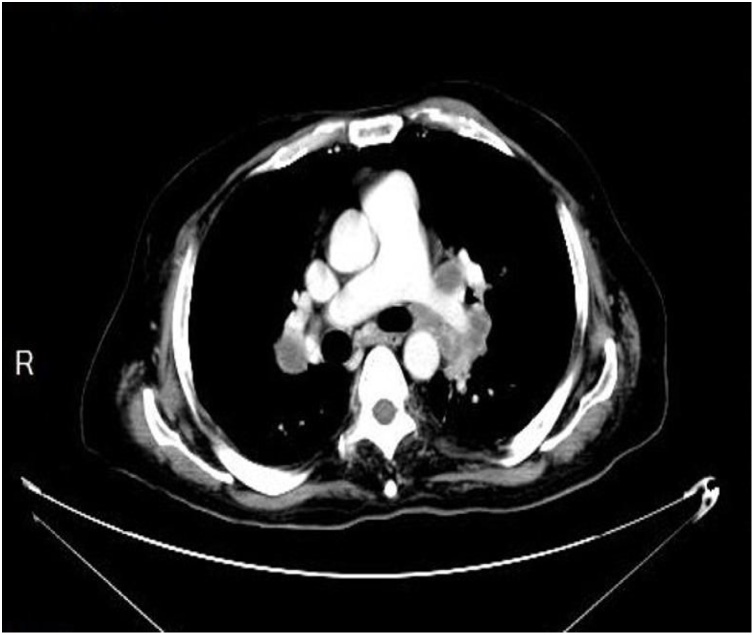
CT scan of the chest with IV contrast shows bilateral hilar and mediastinal enlarged lymph nodes.
